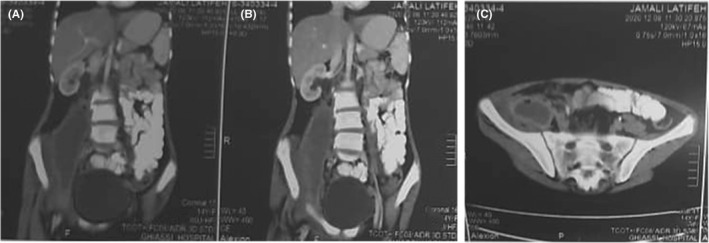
Abdominopelvic CT scan, revealing large collection thorough the entire course of right psoas beginning adjacent to the right kidney up to the right hip. (A), (B) coronal view, (C) axial view
